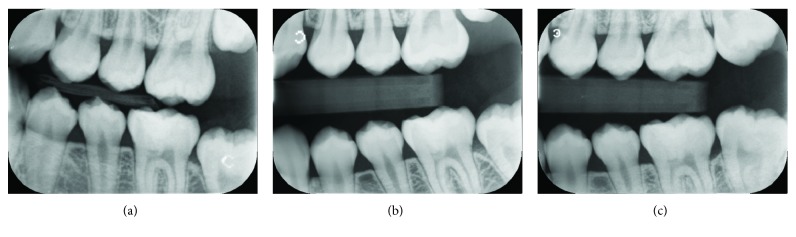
Bitewing radiographs recorded (a) three years postoperative, (b) four years postoperative, and (c) five years postoperative, showing dentine bridge in the left mandibular permanent first molar.
